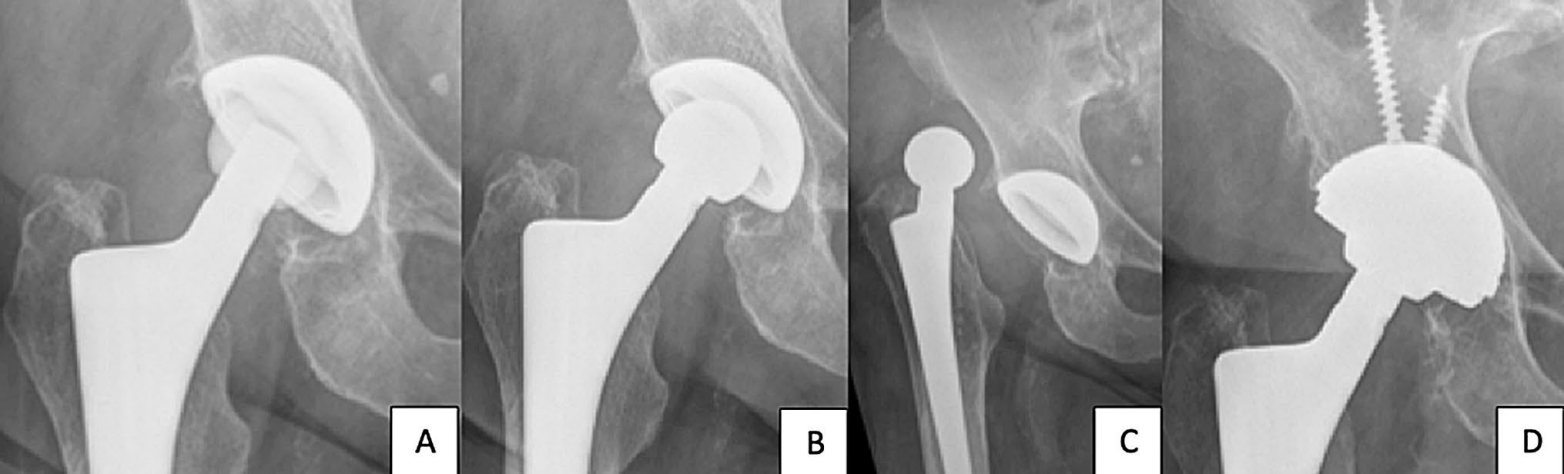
Massiv PE liner wear a without osteolysis around the cup or the greater trochanter. Postoperative x-ray control after revision of the liner. b Dislocation after liner exchange. c Cup revision to a dual mobility liner and cup with additionally screw fixation
